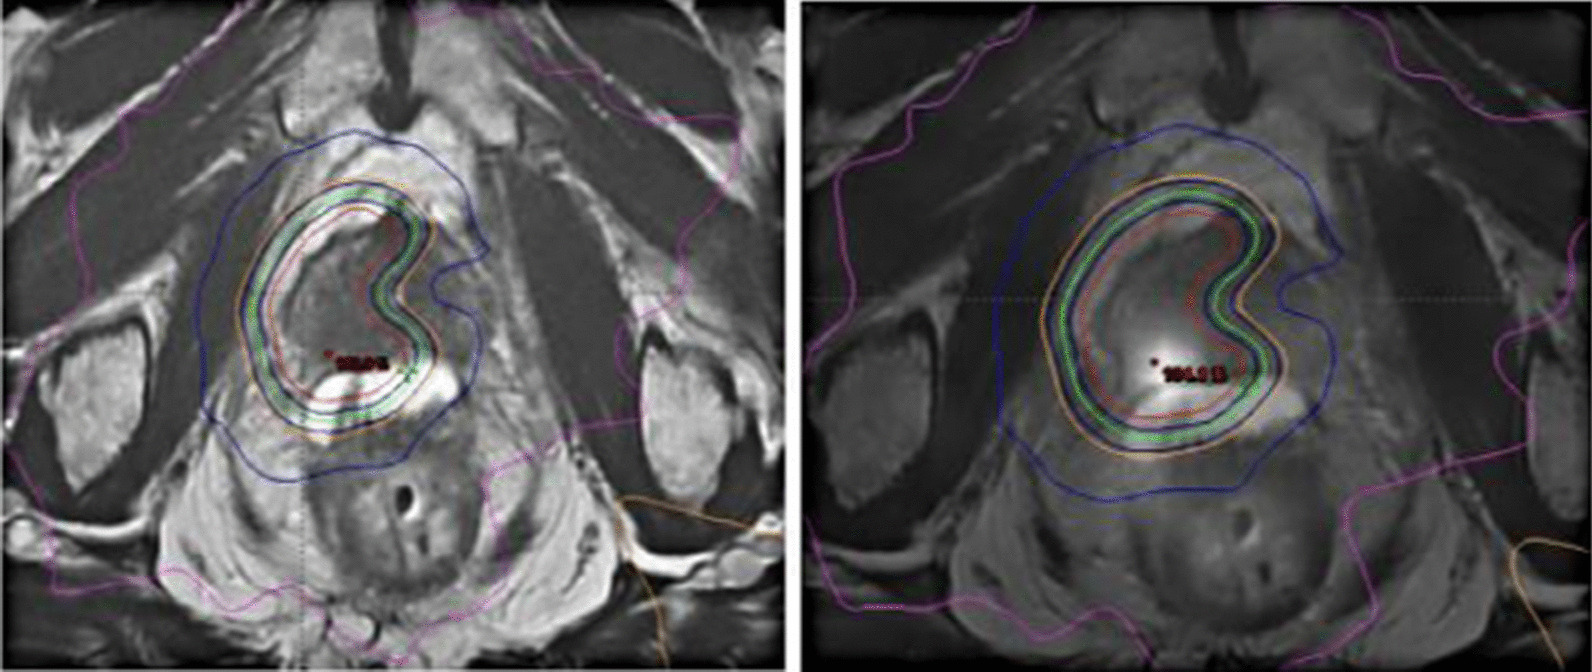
An example of focal re-irradiation using PSMA-PET for target delineation, urethral dose sparing, and a hydrogel rectal spacer
